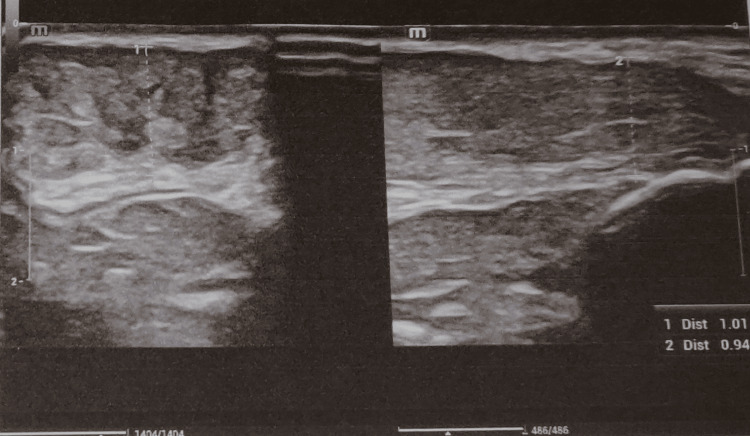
Ultrasound imaging revealed no tendon infiltration, thus differentiating it from a tendinous xanthoma.
